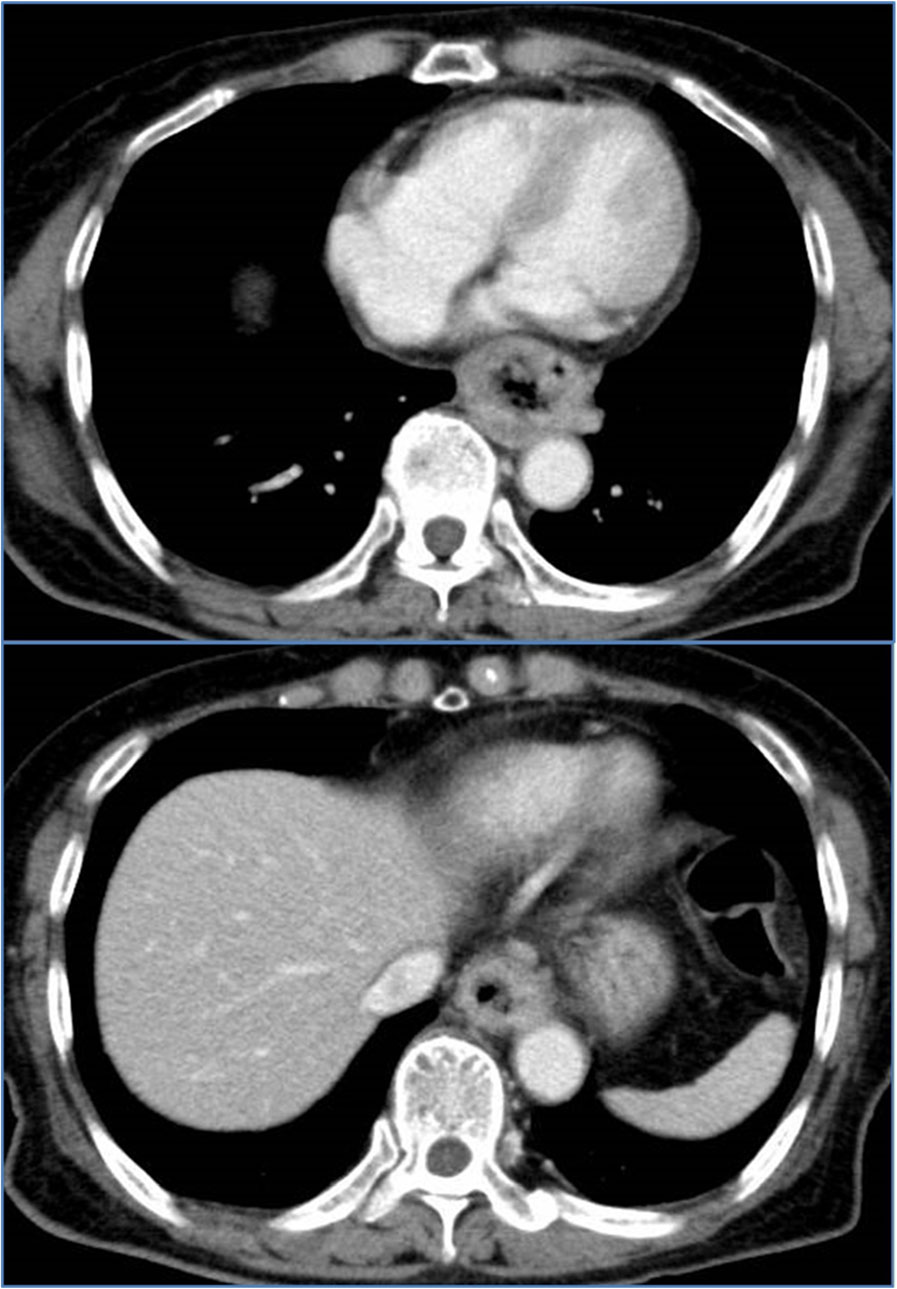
Contrast-enhanced computed tomography findings. Contrast-enhanced computed tomography demonstrated circumferential thickening of the wall of the middle and lower intrathoracic esophagus
